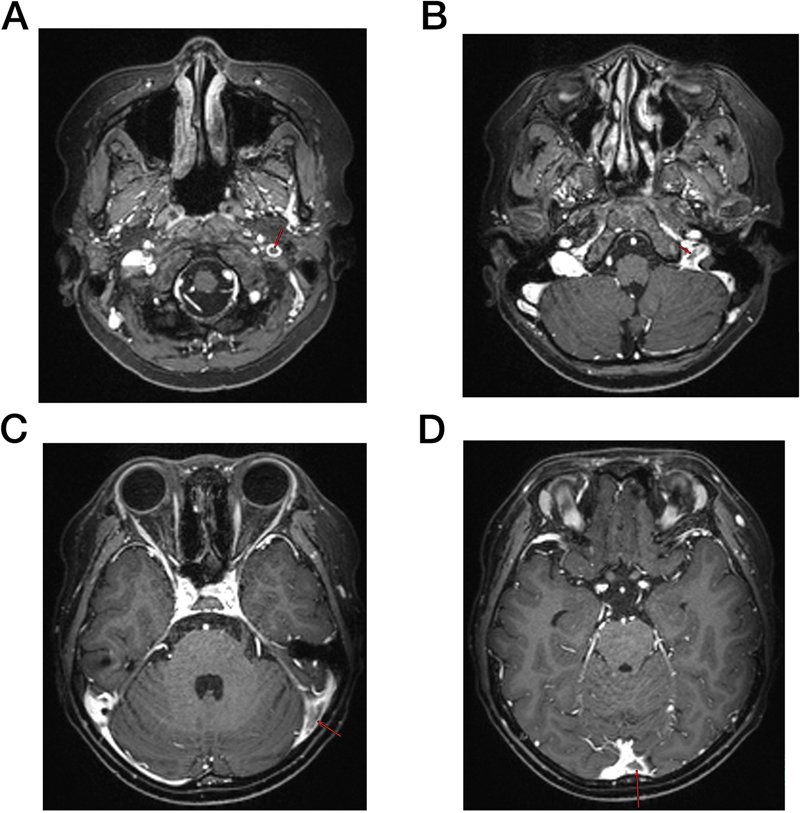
Radiographic data of second thromboses. (
